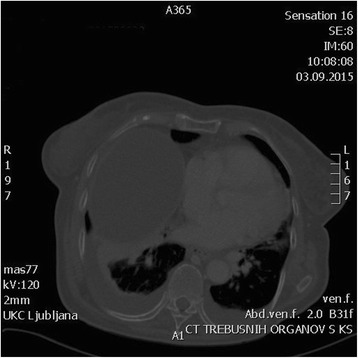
CT scan of recurrent tumor
